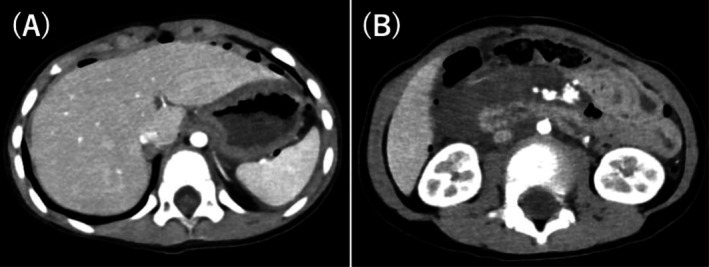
(A) Contrast‐enhanced CT performed at the previous hospital. Free air is observed on the liver surface, suggesting bowel perforation. (B) Contrast‐enhanced CT was performed at the previous hospital. A retroperitoneal hematoma approximately 6 cm in diameter is observed in retroperitoneal Zone 1. No evidence of active extravasation is seen. CT, computed tomography.
